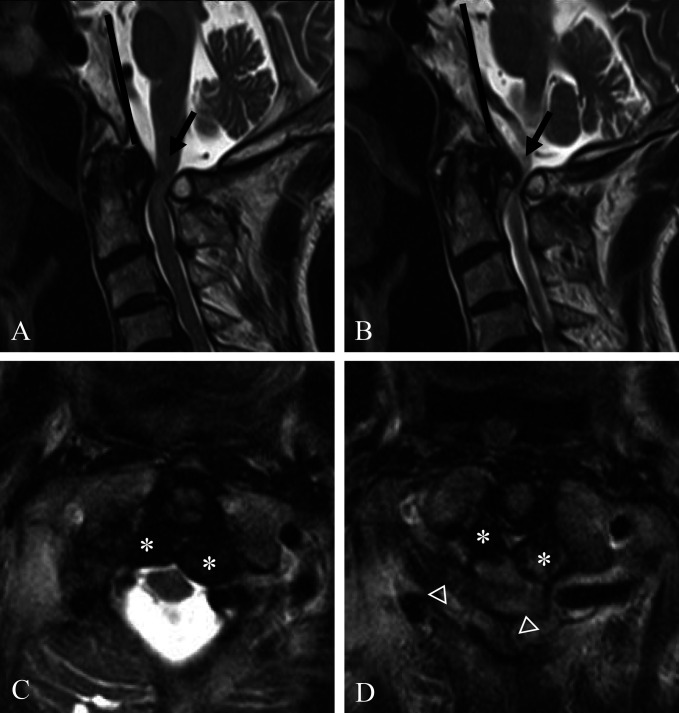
Sagittal MRI (A and B) showing the Wackenheim line (dark lines) behind the dens and severe spinal cord compression by both posttraumatic osteophytes (white arrows) and C1 posterior arch of the C0-C1-C2 joints. Axial MRI (C and D) showing severe spinal cord compression by both posttraumatic osteophytes (asterisks) and the C1 posterior arch (arrowheads).
